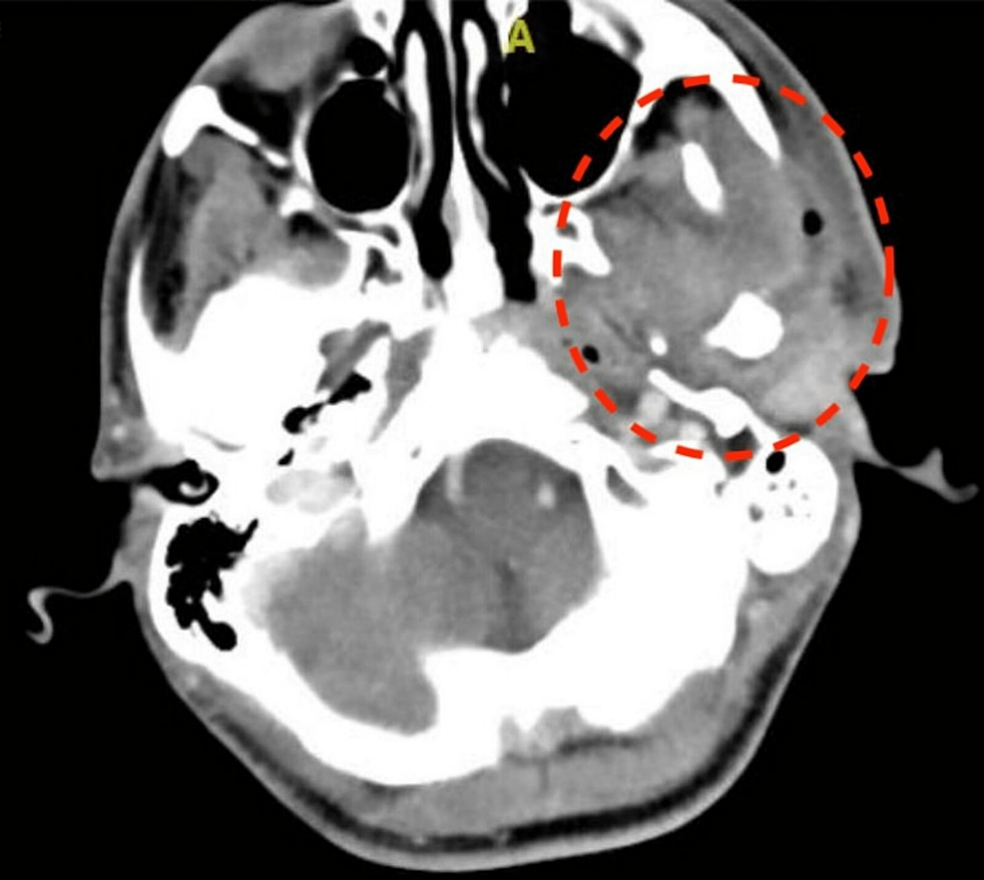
A computerized tomography (CT) scan revealing left parotid capsule erosion and a complicated parotid fistula
